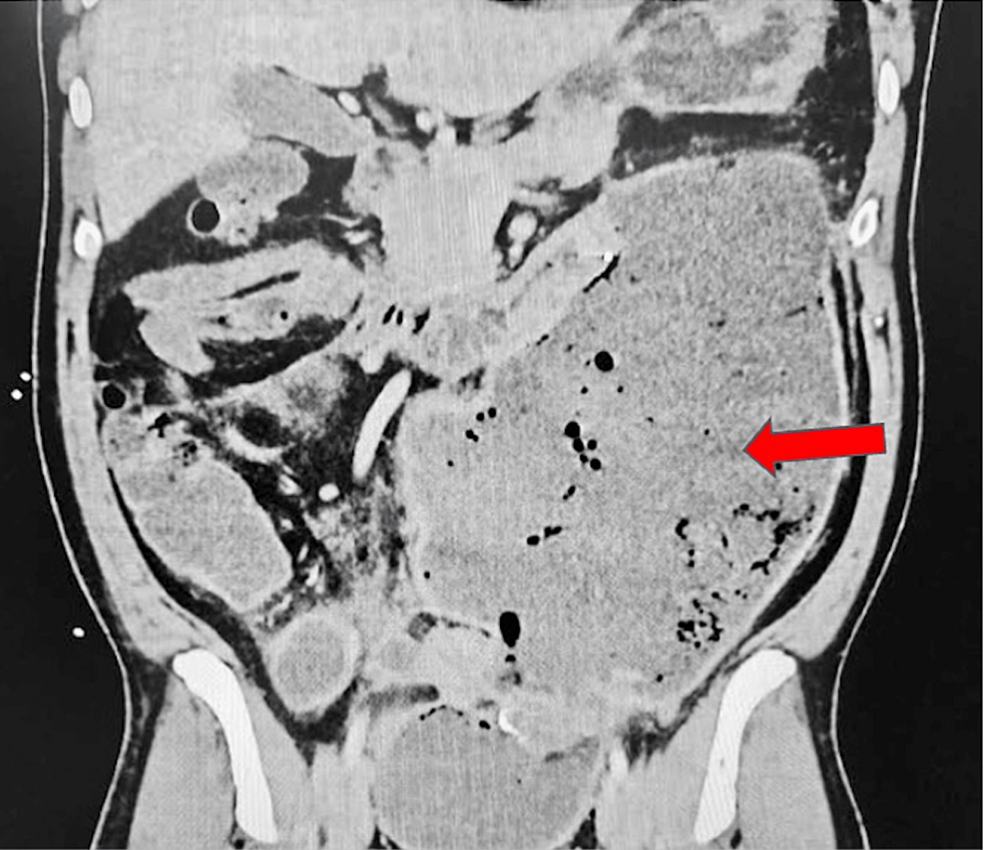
CT with contrast of abdomen and pelvis CT with contrast of the abdomen and pelvis demonstrated a large fluid and gas collection centered in the left abdomen (red arrow), which measured approximately 18 x 9.5 centimeters at its widest margins and extended approximately 26 centimeters craniocaudally.
